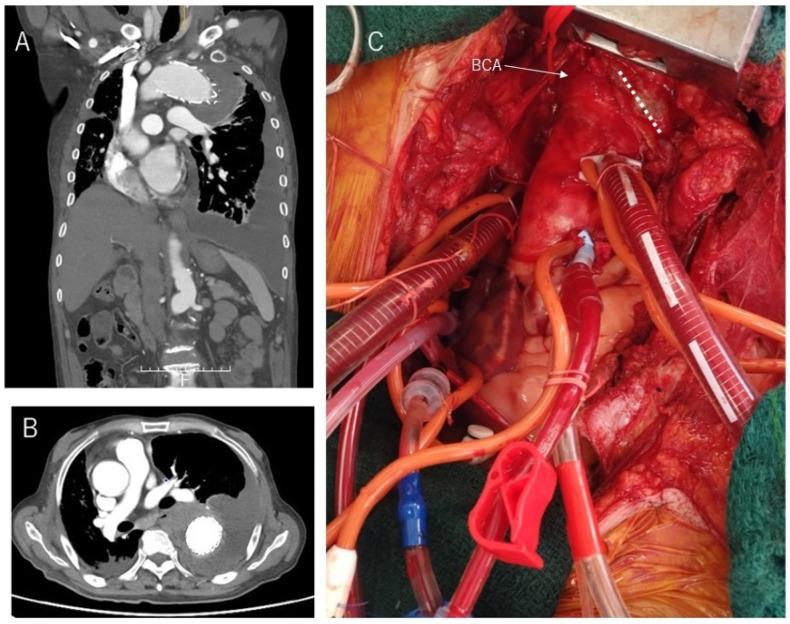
AICT performed via right thoracotomy in a patient with aneurysmal enlargement after TEVAR. (A) Preoperative sagittal computed tomography demonstrating aneurysmal expansion of the distal arch secondary to a type I endoleak after previous TEVAR, with a proximal stent-graft “beak sign” indicating incomplete apposition in the sharply angulated arch. (B) Axial CT image showing the enlarged distal arch aneurysm and the malapposed proximal stent graft. (C) Intraoperative view through a right thoracotomy approach. The dotted line indicates the aortotomy closure (suture) line, which was created adjacent to the brachiocephalic artery (BCA).
